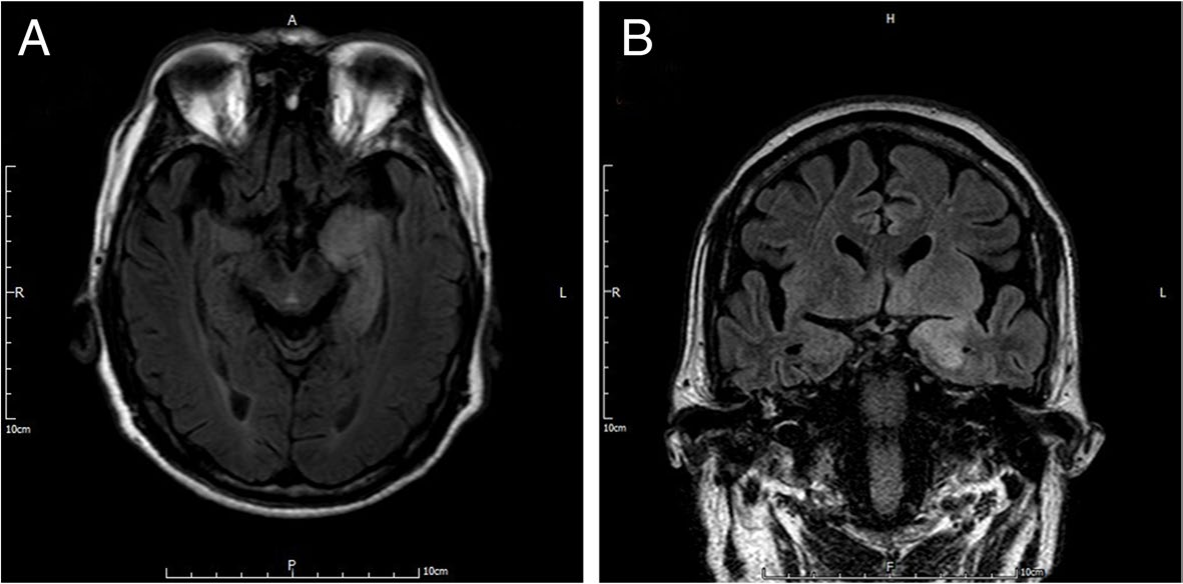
MRI sequences on the second admission. These images demonstrated new lesion which was hyperintensity on FLAIR sequences in the left hippocampus
